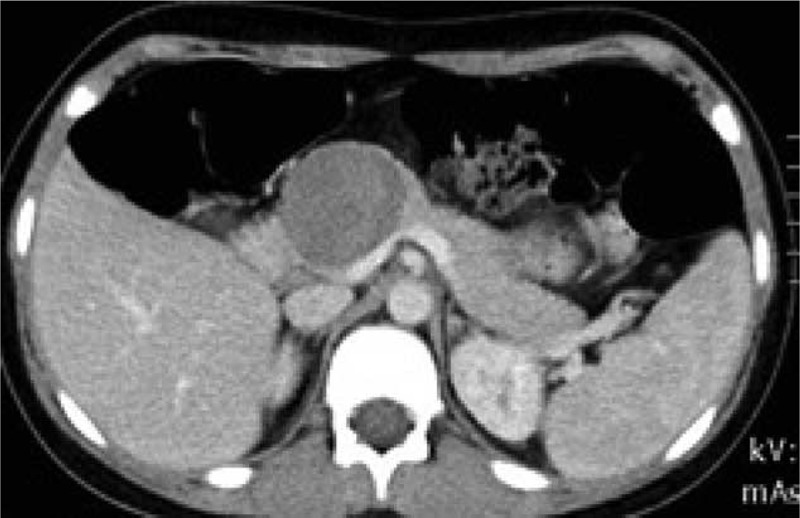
Computed tomography (CT) scan: the round shape mass located in the head of the pancreas.
